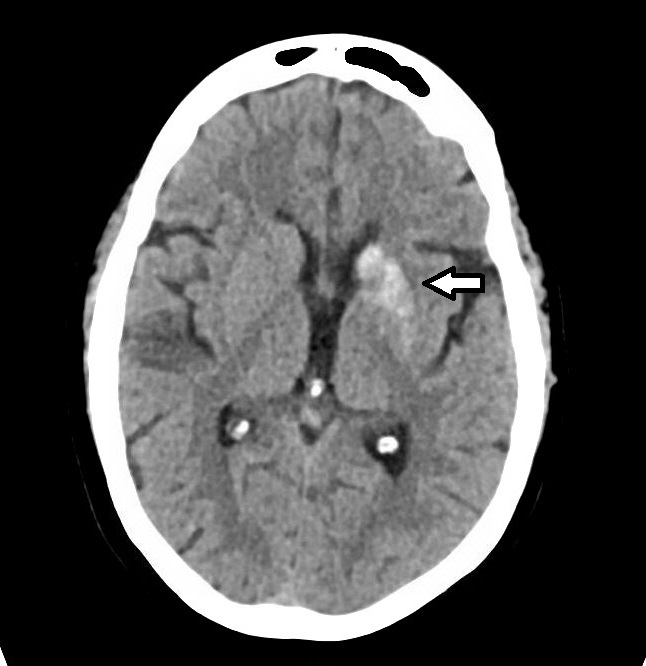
Computed tomography head imaging, which shows hyperintensity (arrow) in left caudate nucleus concerning for potential hemorrhagic stroke.
